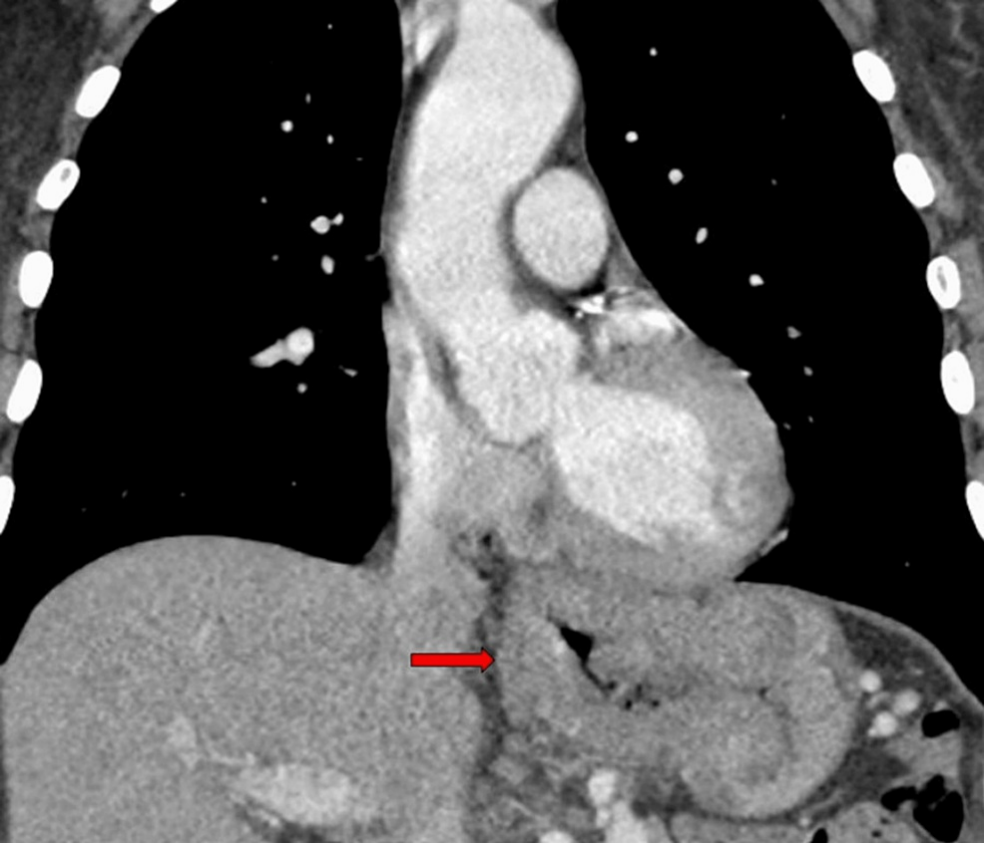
CT image showing primary tumor in coronal plane (red arrow). CT, computed tomography
